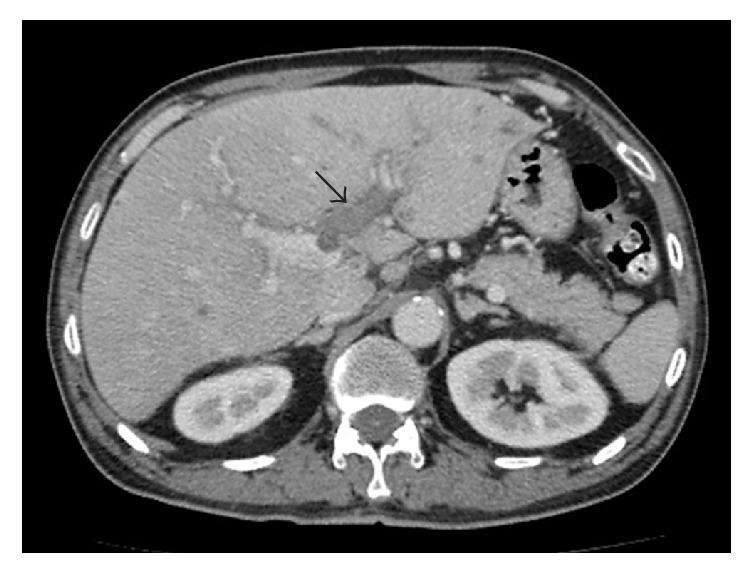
Axial computed tomography (CT) scan (portal venous phase) shows thrombosed left portal vein (arrow). Multiple small periportal vessels, which represent dilated collateral veins, which is the so-called cavernous transformation. Left portal vein thrombosis caused transient hepatic attenuation differences (THAD) between the left and right lobes of the liver.
